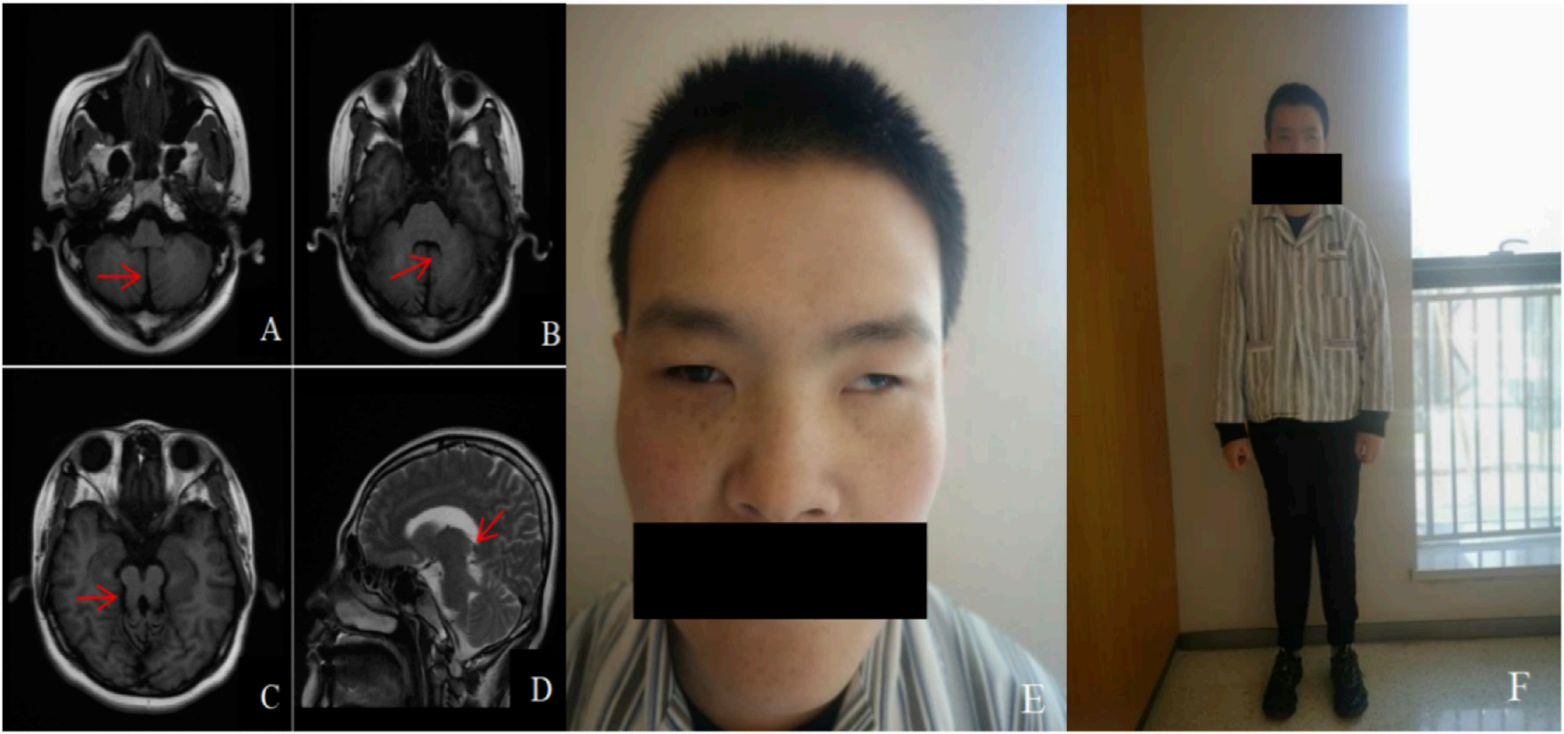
Cranial MRI and facial and systemic manifestations of patient: (A). Axial view T1WI shows the “midline cleft” sign (red arrow); (B). Axial view T1WI shows the “batwing” fourth ventricle (red arrow); (C). Axial view T1WI shows the “molar sign” (red arrow); (D). Sagittal view T2WI shows the thickened and elongated pedunculus cerebellaris superior, which runs in an anterior-posterior direction (red arrow); (E), (F). Special face, featuring blepharophimosis, wide inner canthal distance, mild ptosis, severe positive epicanthus, high palatal arches, exotropia, hypotrichosis of the beard and face, and inconspicuous prominentia laryngea; Fair skin with no fine hair all over, shorter upper and lower limbs, and instability of gait.
